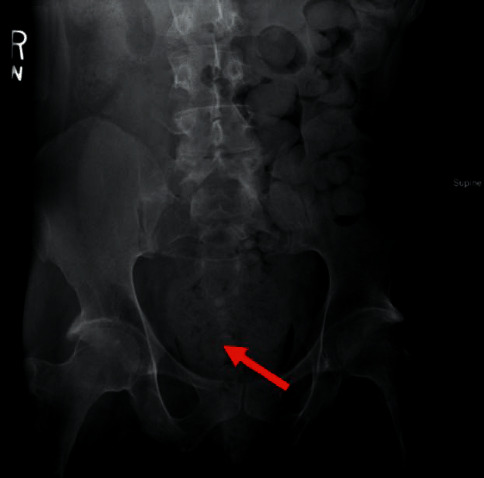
Abdominal X-ray radiography demonstrating fecaloma in a 34-year-old patient with intellectual and developmental disabilities and chronic constipation. The image shows a 10.2 cm fecaloma (red arrow) located in the rectum, with the presence of a high colonic stool burden proximally.
